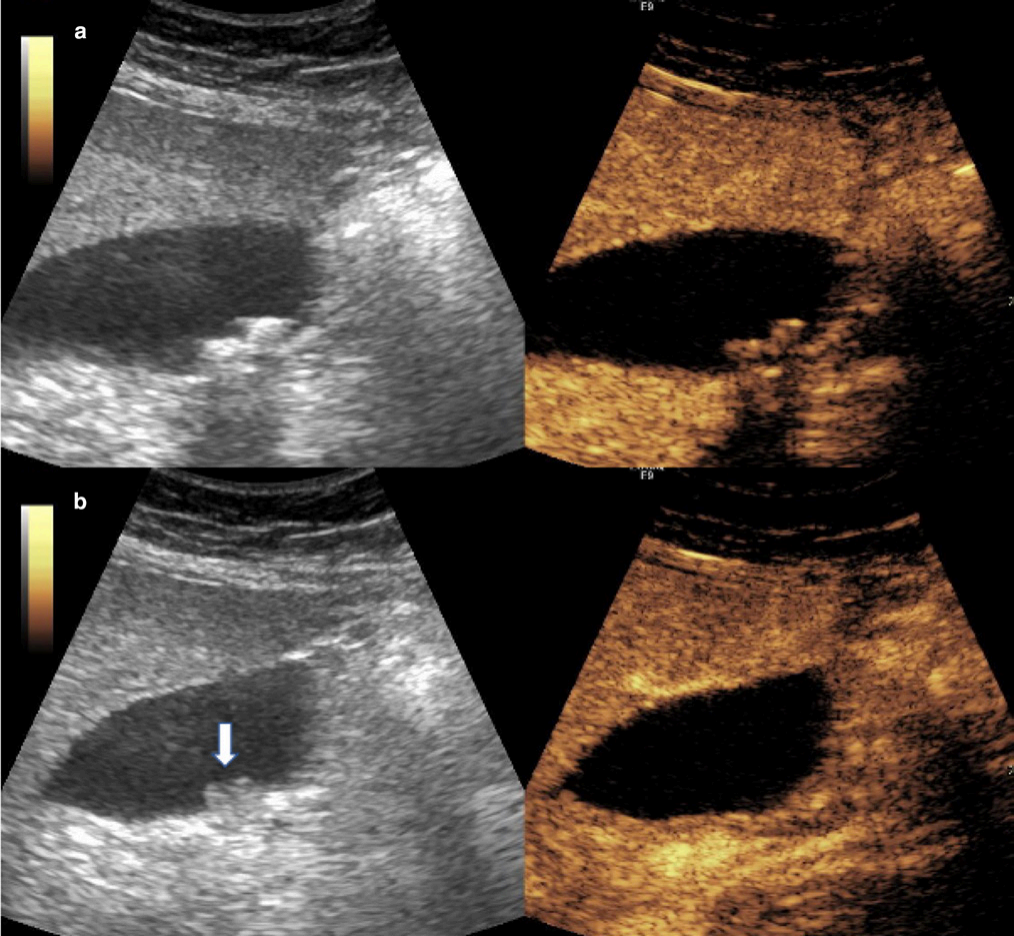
Contrast-enhanced ultrasound images for assessment of indeterminate gallbladder findings and problem solving. (a) Typical appearance of gallstones within the gallbladder lumen causing posterior acoustic shadowing. (b) A polypoid lesion at the posterior wall of the gallbladder on the greyscale image does not demonstrate enhancement on the corresponding contrast-enhanced image and is therefore in keeping with adherent sludge or pseudopolyp (white arrow).
